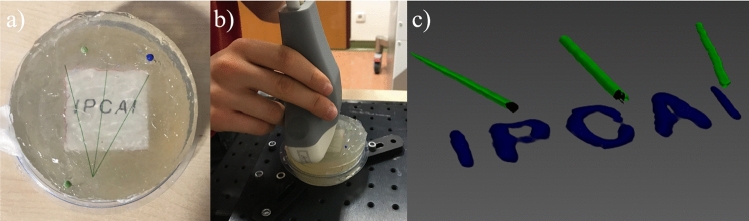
The experimental setup and results of the *feasibility demonstration* experiment. The IPCAI logo printed on paper is placed underneath a gel pad on top of which the optical pattern is fixed and some ultrasound gel is added (a). A freehand PA scan is acquired (b), which results in a fully image-based and clear reconstruction of the IPCAI logo
